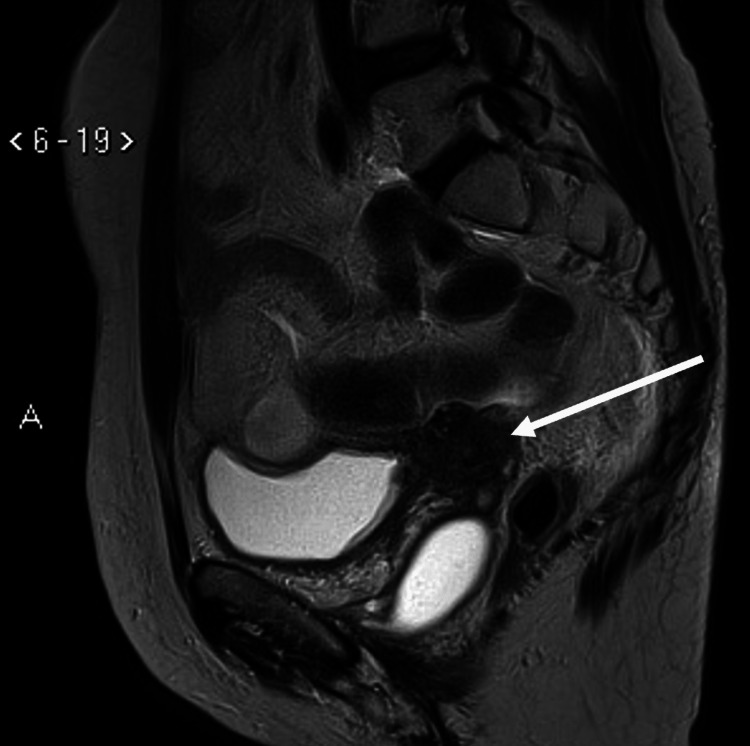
Magnetic resonance imaging demonstrated a solid mass measuring 6.3 x 2.2 x 3.0 cm, with a retractable aspect in the vaginal dome, presenting a low signal in T2 and enhanced by contrast.
